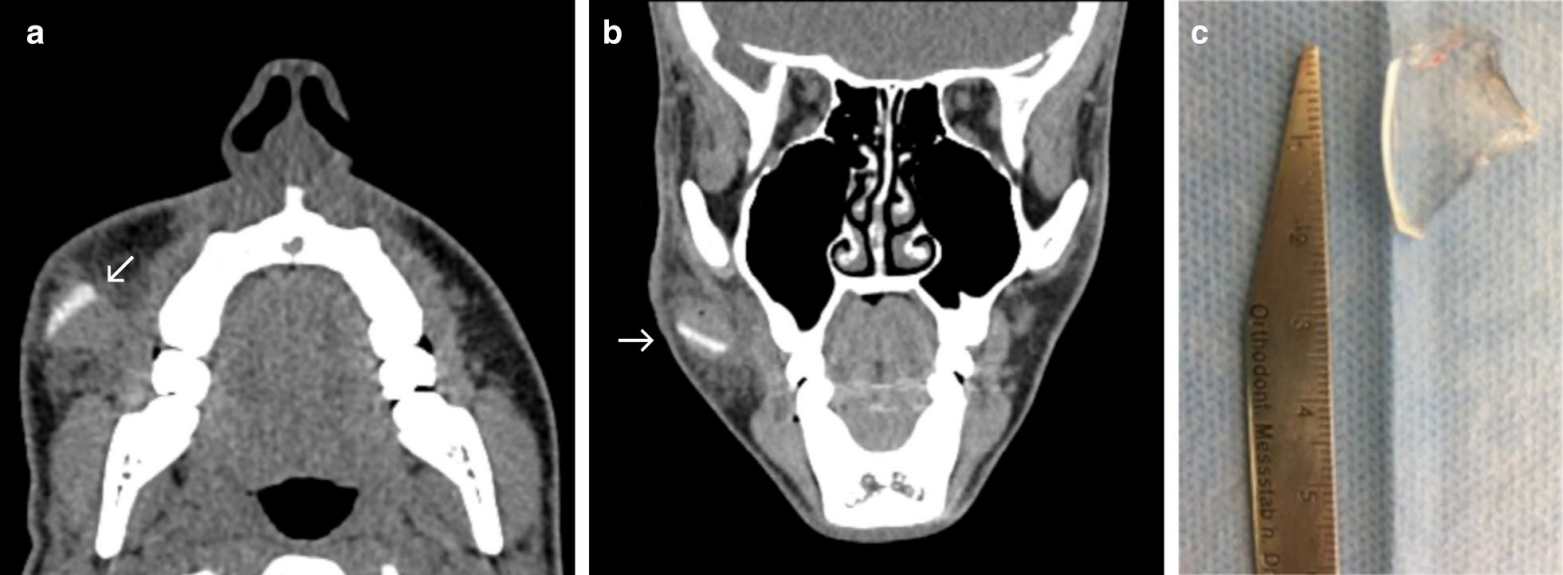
Broken ophthalmic lens. Axial (a) and coronal (b) CT images showing a hyperdense foreign object (arrow) in the right buccal area surrounded by haematoma. Photograph of a translucent piece of glass after surgical retrieval (c)
